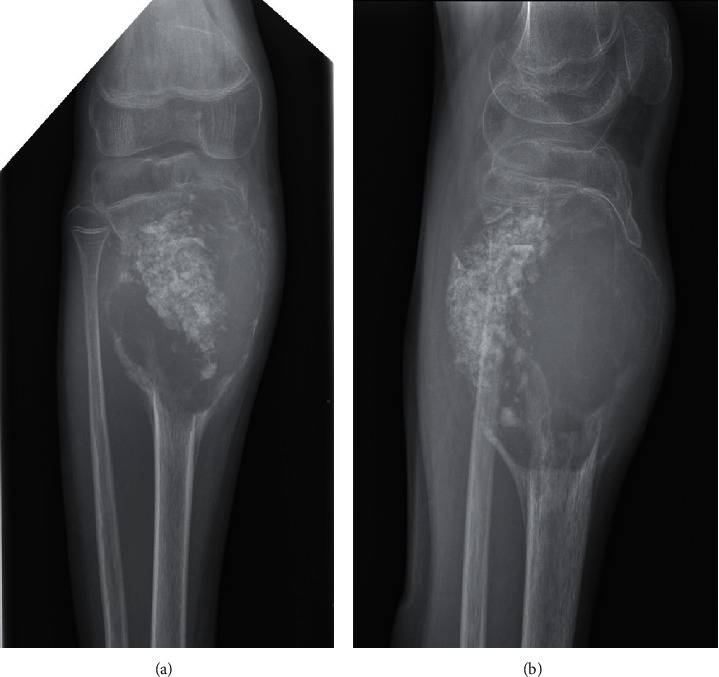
Anteroposterior (a) and lateral (b) plain radiographs of the right lower leg demonstrating a recurrent ABC after initial detailed curettage and allogenic bone grafting.
